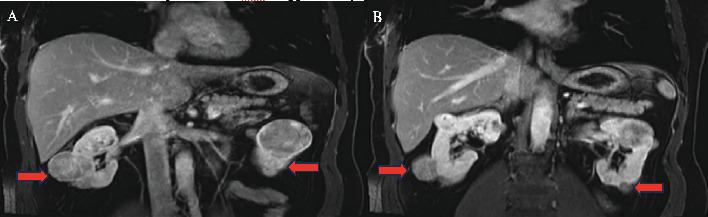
(A, B) CT scan showing bilateral renal tumors.
